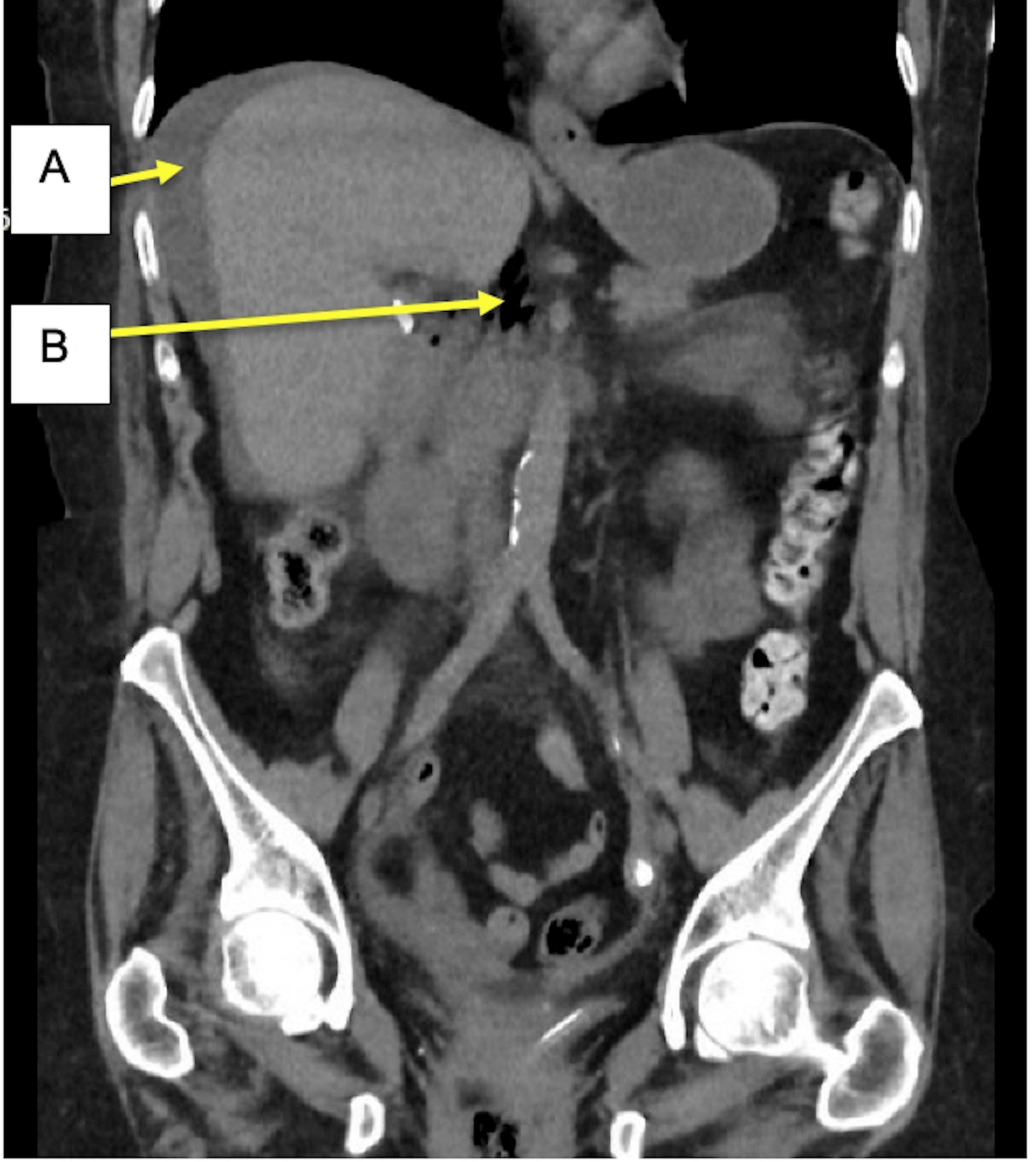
Coronal slice of CT KUB demonstrating a moderate volume of perihepatic intra-abdominal free fluid (A) and locules of extraluminal air (B) in proximity to the duodenum KUB, kidneys, ureters, and bladder
